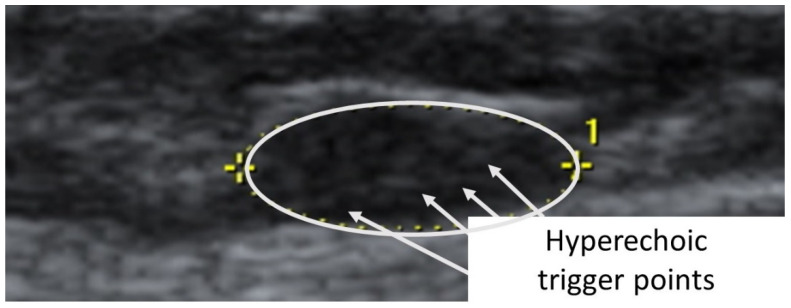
Detailed ultrasound imaging assessment of the left upper trapezius muscle showing a collection of small hyperechoic (hypoperfused) “TrP speckles” within the contracture knot.
